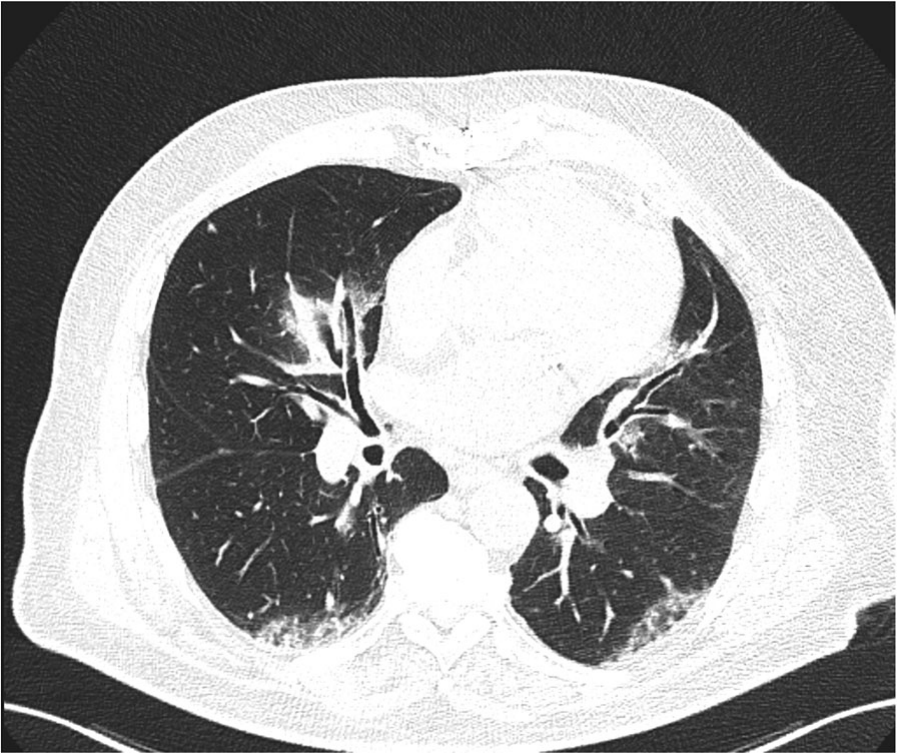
Sixty-nine-year-old man admitted at the intensive care unit with dyspnea. Axial chest computed tomography demonstrates ground-glass opacities with peribronchovascular distribution
